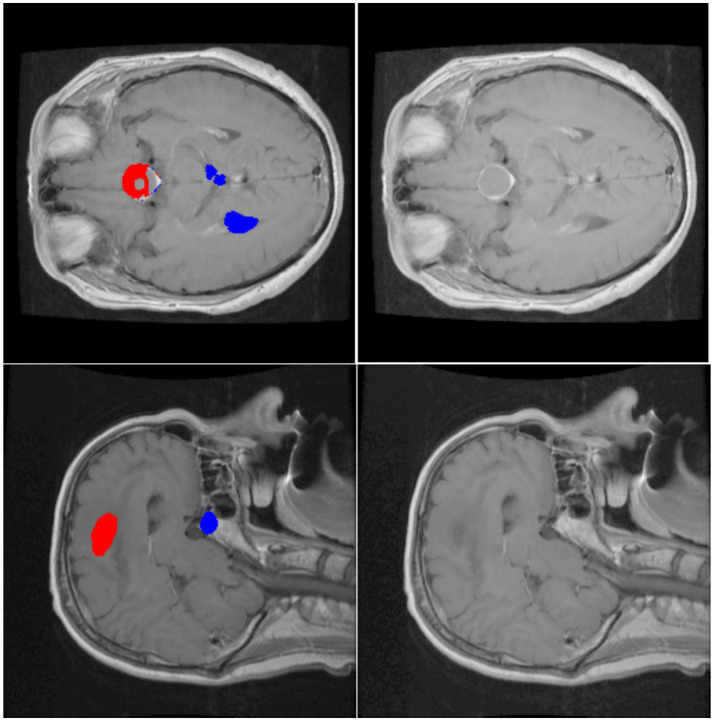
Examples of MRI with FPs and FNs.
